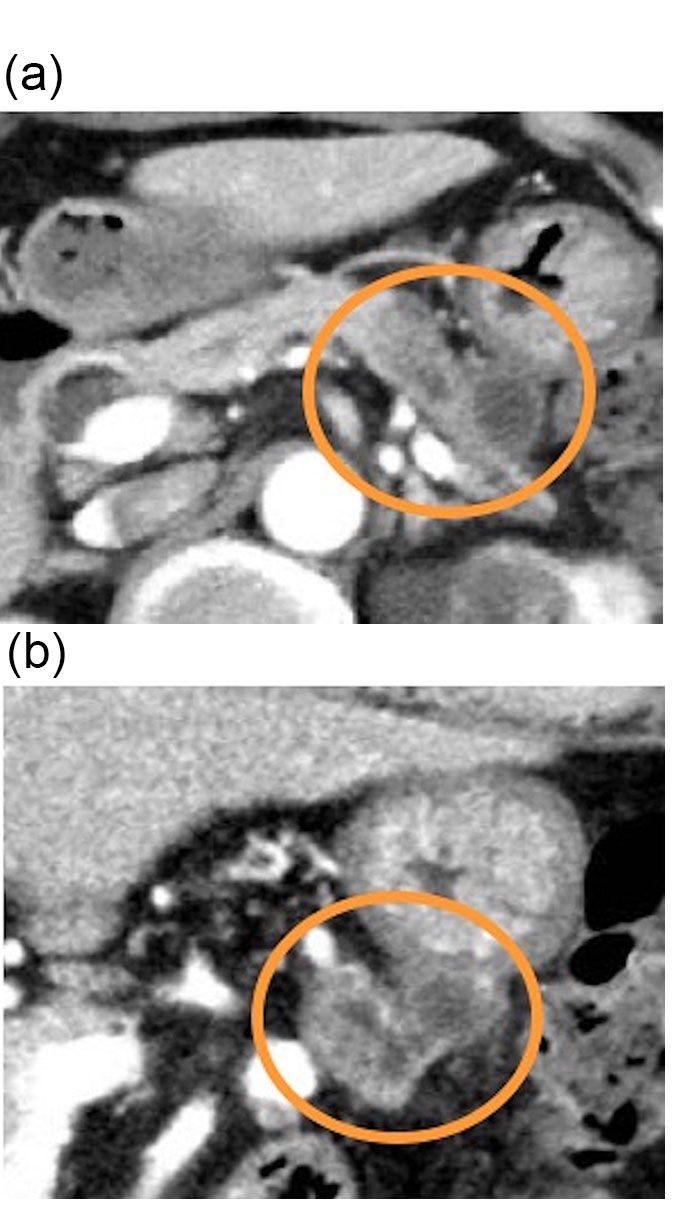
(a) Axial image and (b) coronal image computed tomography revealed a new 18‐mm‐diameter cystic lesion with a thick wall between the pancreas and the stomach (circle)
